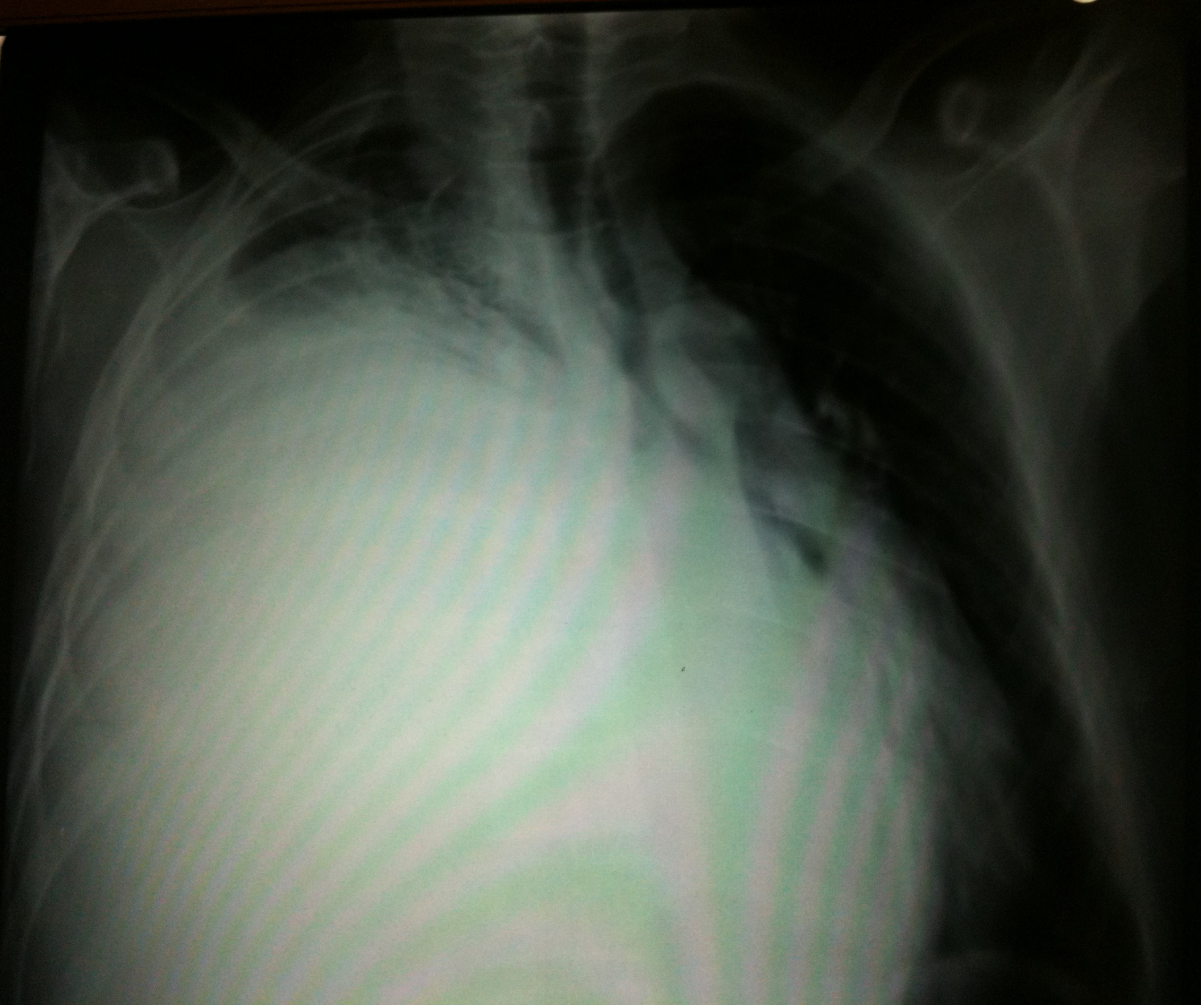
Chest radiograph (postero-anterior view) shows the presence of a right-sided opaque hemithorax.
